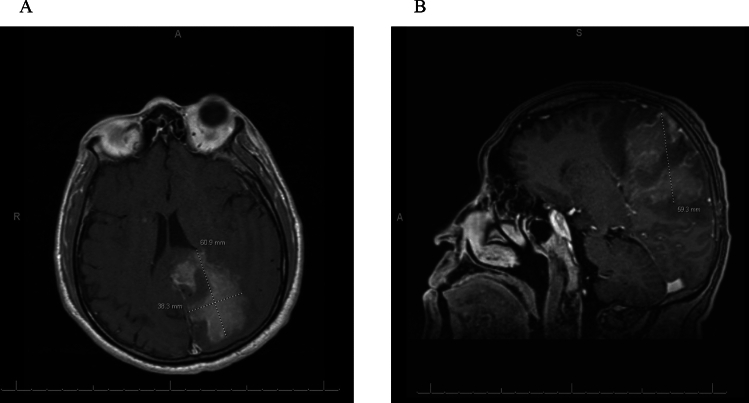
Day 11 brain imaging. A Axial T1 MRI with contrast depicting 60.9 × 38.3 mm mass-like lesion, favoring glioma or glioblastoma. B Sagittal T1 MP-RAGE with contrast redemonstrating mass-like lesion, favoring glioma or glioblastoma
